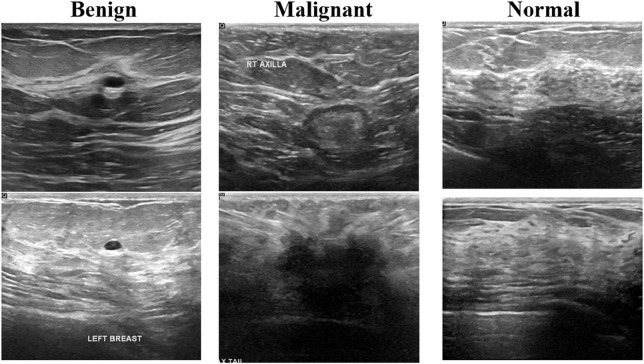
Ultrasound images of breast cancer from the selected dataset.
